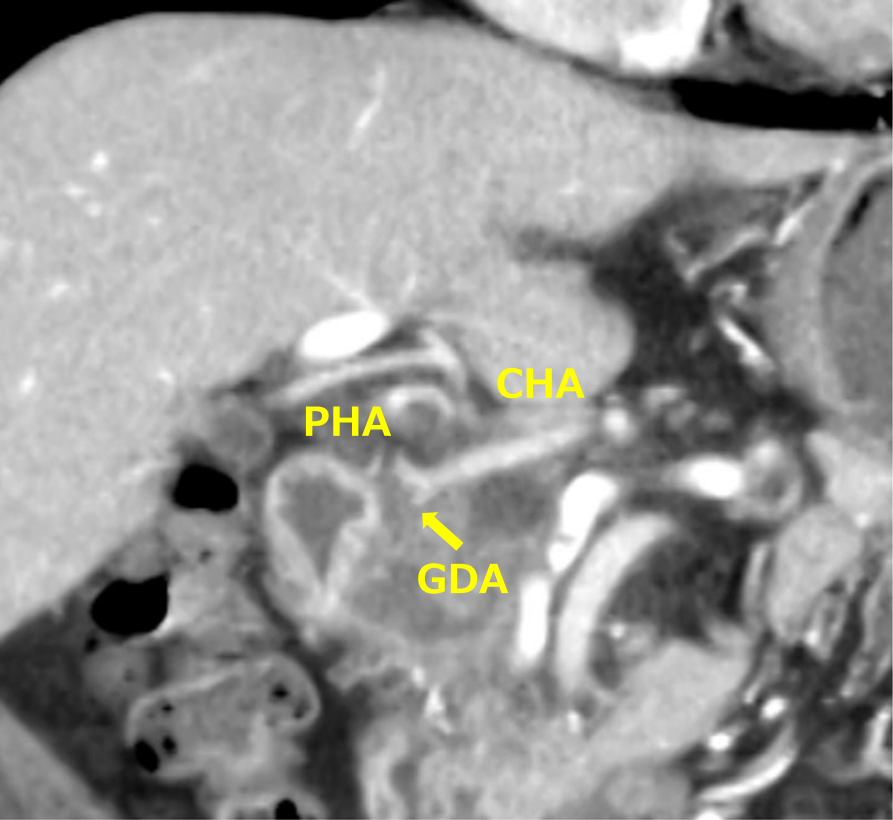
Contrast-enhanced CT revealed a low-density tumor in the pancreatic head, which was invading the CHA and the root of the GDA. CHA, common hepatic artery; PHA, proper hepatic artery; GDA, gastroduodenal artery
